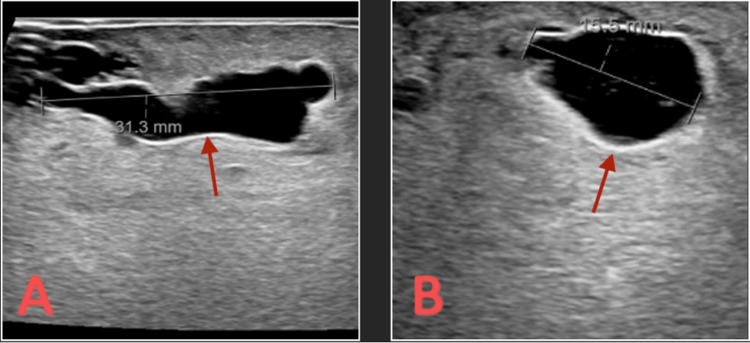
(A) Soft tissue ultrasound showing 31.1 mm fluid collection in the right glute. (B) Soft tissue ultrasound showing 15.5 mm fluid collection in the right glute.
